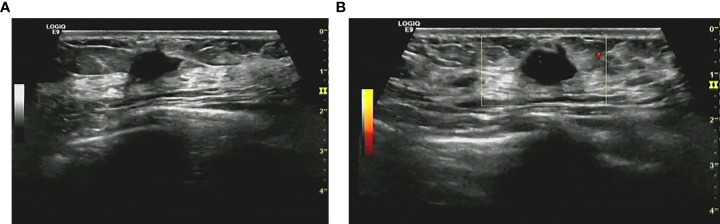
(A) Conventional ultrasonography demonstrated an irregular hypoechoic breast lesion without calcification categorized as BI-RADS 4b. (B) Only few vascular spots were seen in the lesion.
